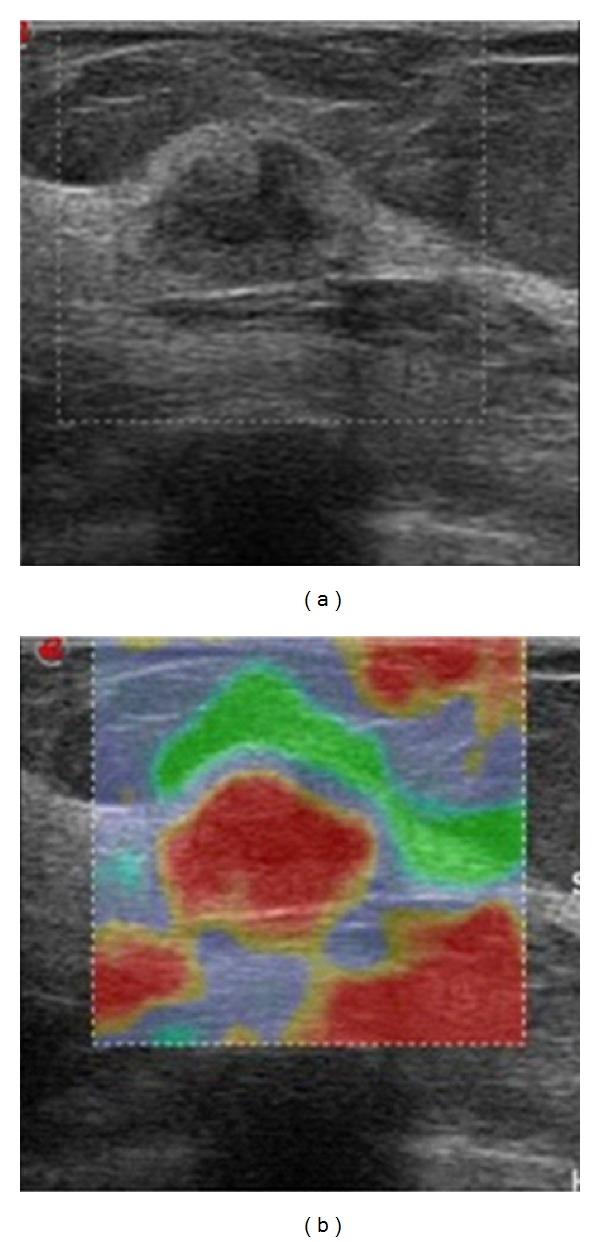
Comparative B-mode ultrasound image of an unspecified lesion which, on the elastographic map, corresponds to an increased hardness lesion, shown in red. It corresponded to invasive ductal carcinoma.
